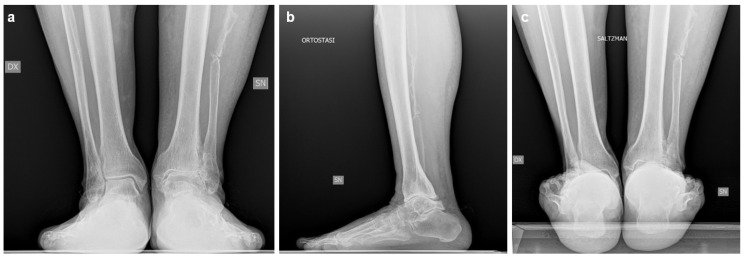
Preoperative weight-bearing radiographs of Case two: (a) anteroposterior view; (b) lateral view; (c) Saltzman view.
